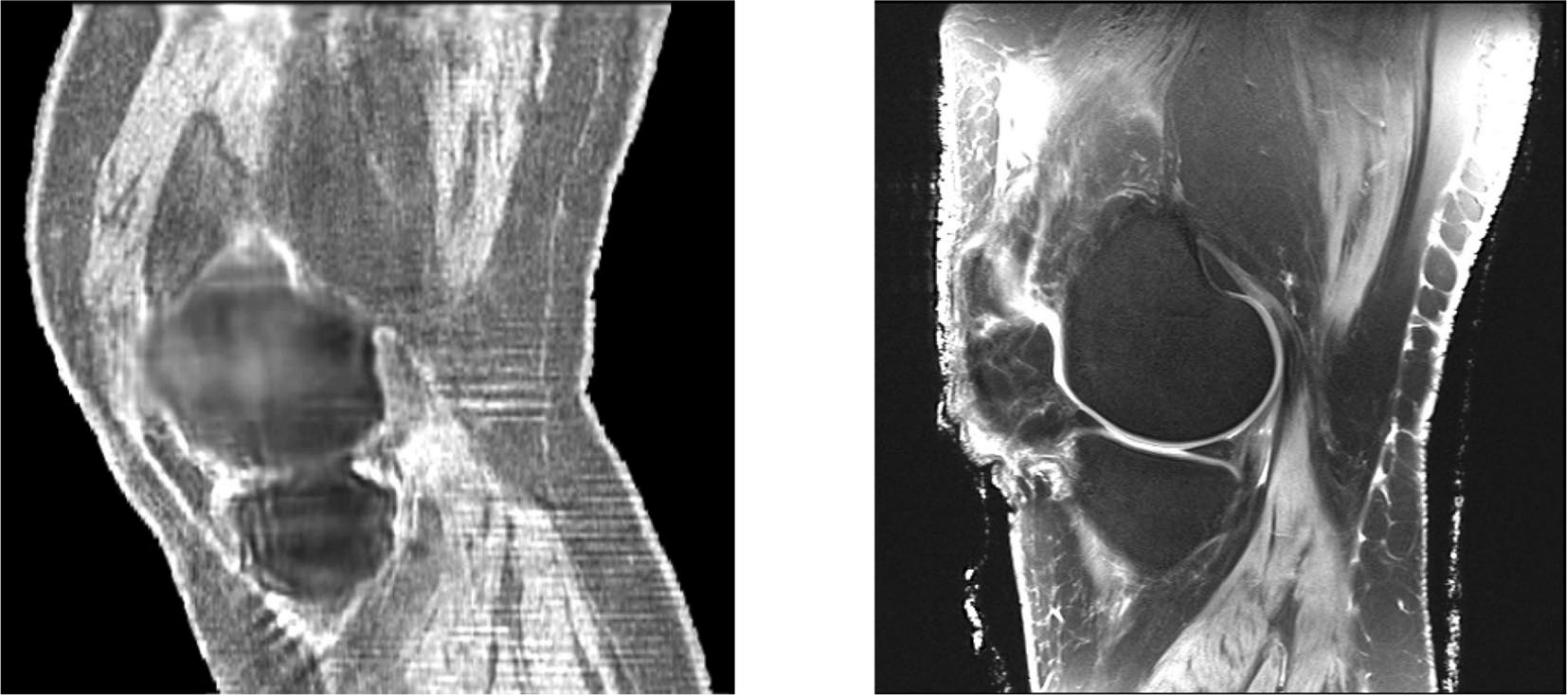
Sagittal view of knee: QTUS image on the left, MR image on the right. Note the close correspondence in the muscle tissue, bone, cartilage and patellar tendon and Hoffa’s fat pad. The bone is delineated.
